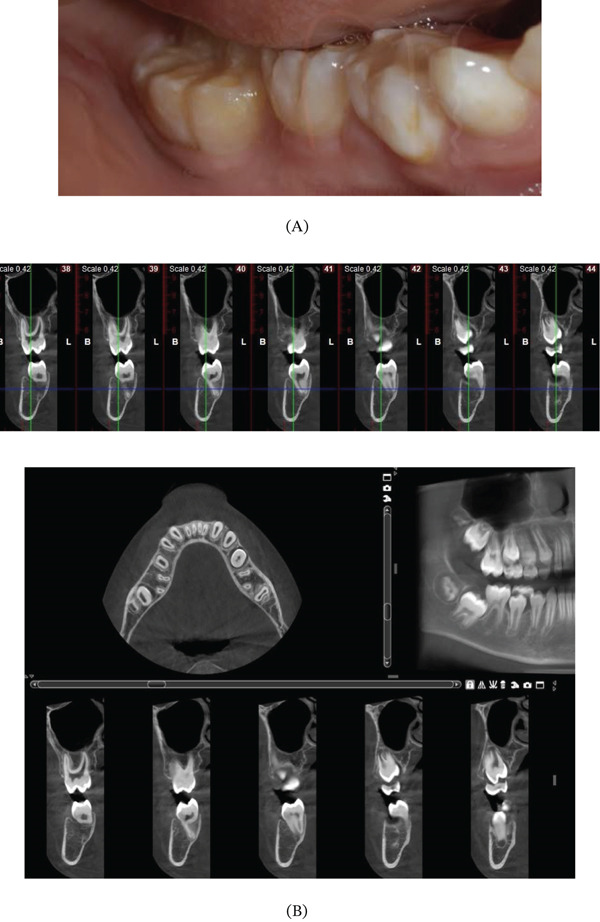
Final photos of the follow‐up: (A) Clinical view at 3 months; (B) at 1 year, a CBCT of the area confirms complete resolution.
